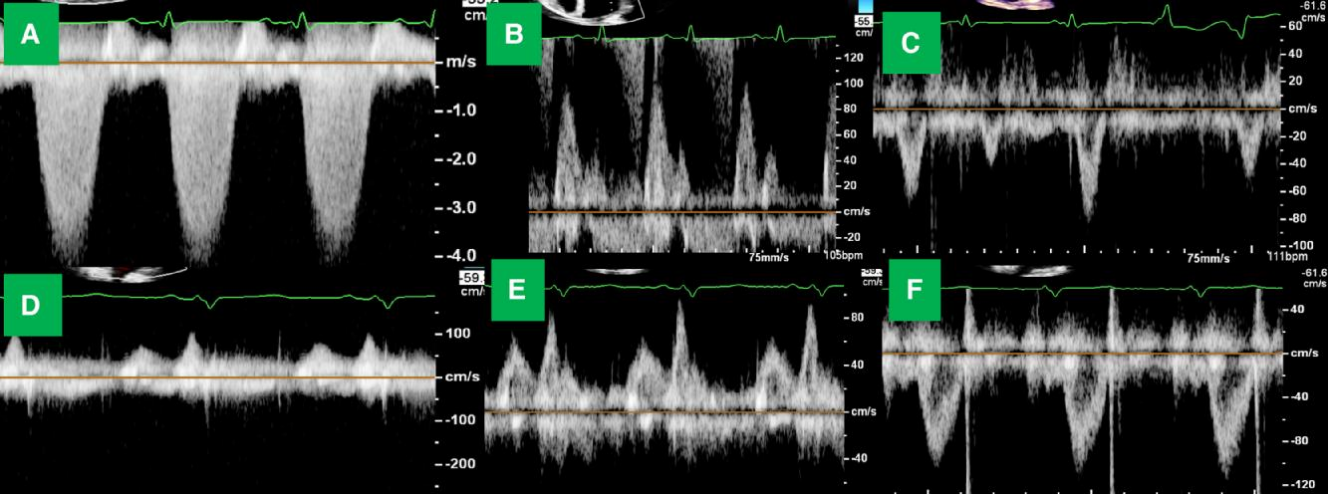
Patient 2 non-invasive haemodynamics. Admission: (*A*) mitral continuous wave Doppler showing triangular early peaking jet, (*B*) mitral inflow pattern E>>A, (*C*) severely diminished left ventricular outflow tract velocity time integral 6 months later: (*D*) absent mitral continuous wave Doppler signal, (*E*) mitral inflow with E/A reversal and relatively small E-wave, (*F*) normal left ventricular outflow tract velocity time integral.
